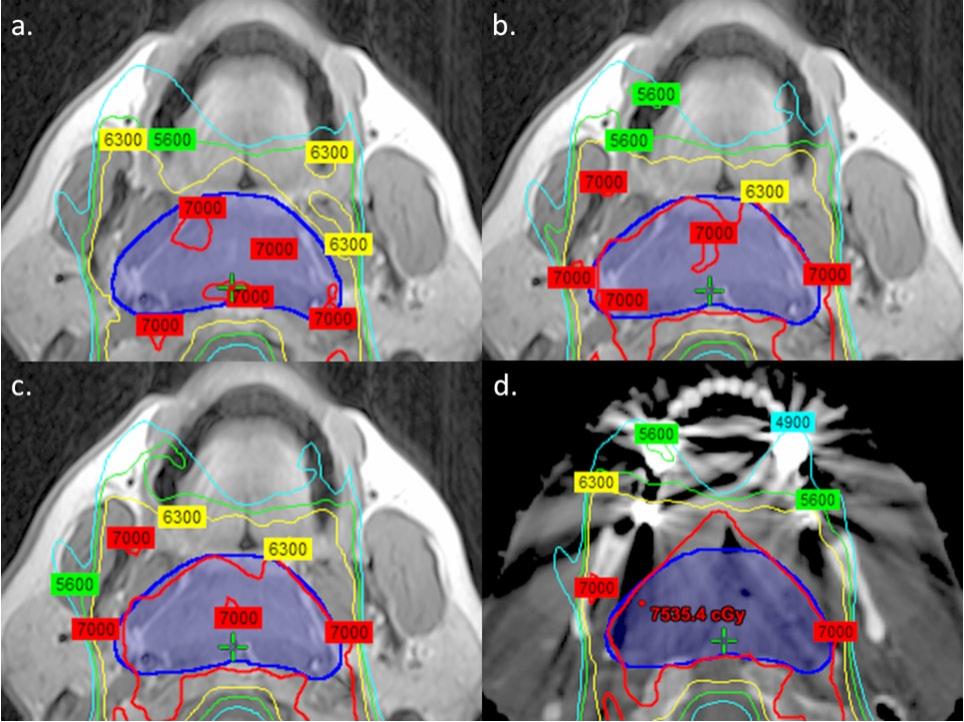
Comparison of isodose distributions between (a) ${\text{MRI}}_{W}$‐based, (b) ${\text{MRI}}_{W\;+\;B}$‐based, (c) ${\text{MRI}}_{W\;+\;B\;+\;A}$‐based, and (d) CT‐based inverse‐optimized IMRT plans for Patient 3. Images were obtained at the identical axial level based on CT‐MRI registration. Isodose distributions were calculated on CT with heterogeneity correction to standardize comparisons.
